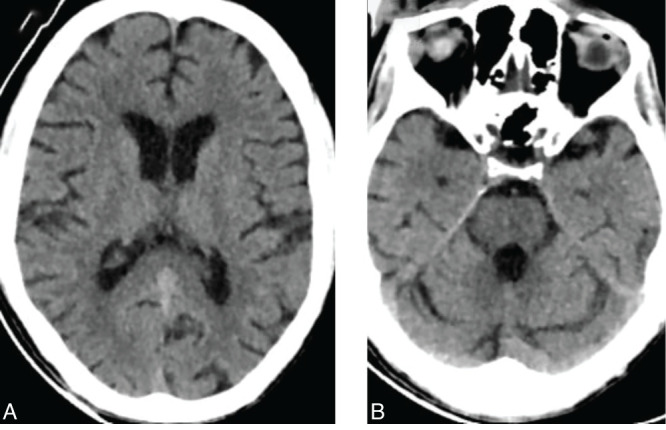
Axial (A and B) non-contrast CT images of the head reveal no evidence of abnormalities in the cerebral white matter, basal ganglia, or pons.
